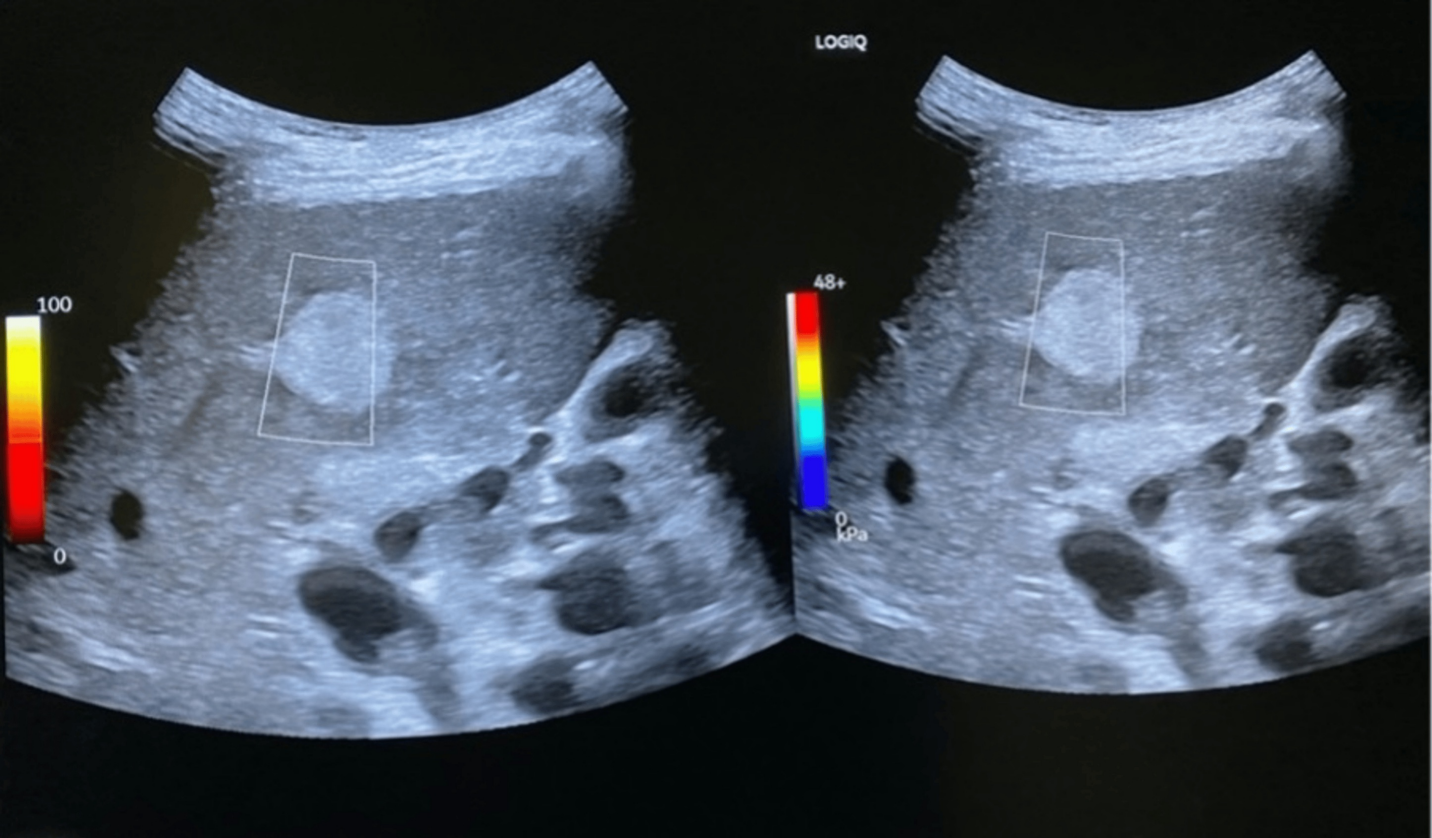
2D-SWE in malignant lesion in the liver parenchyma (lesion stiffness 24 kPa) The ultrasound images shown are part of our team's original work and were obtained by the authors as part of this study.
